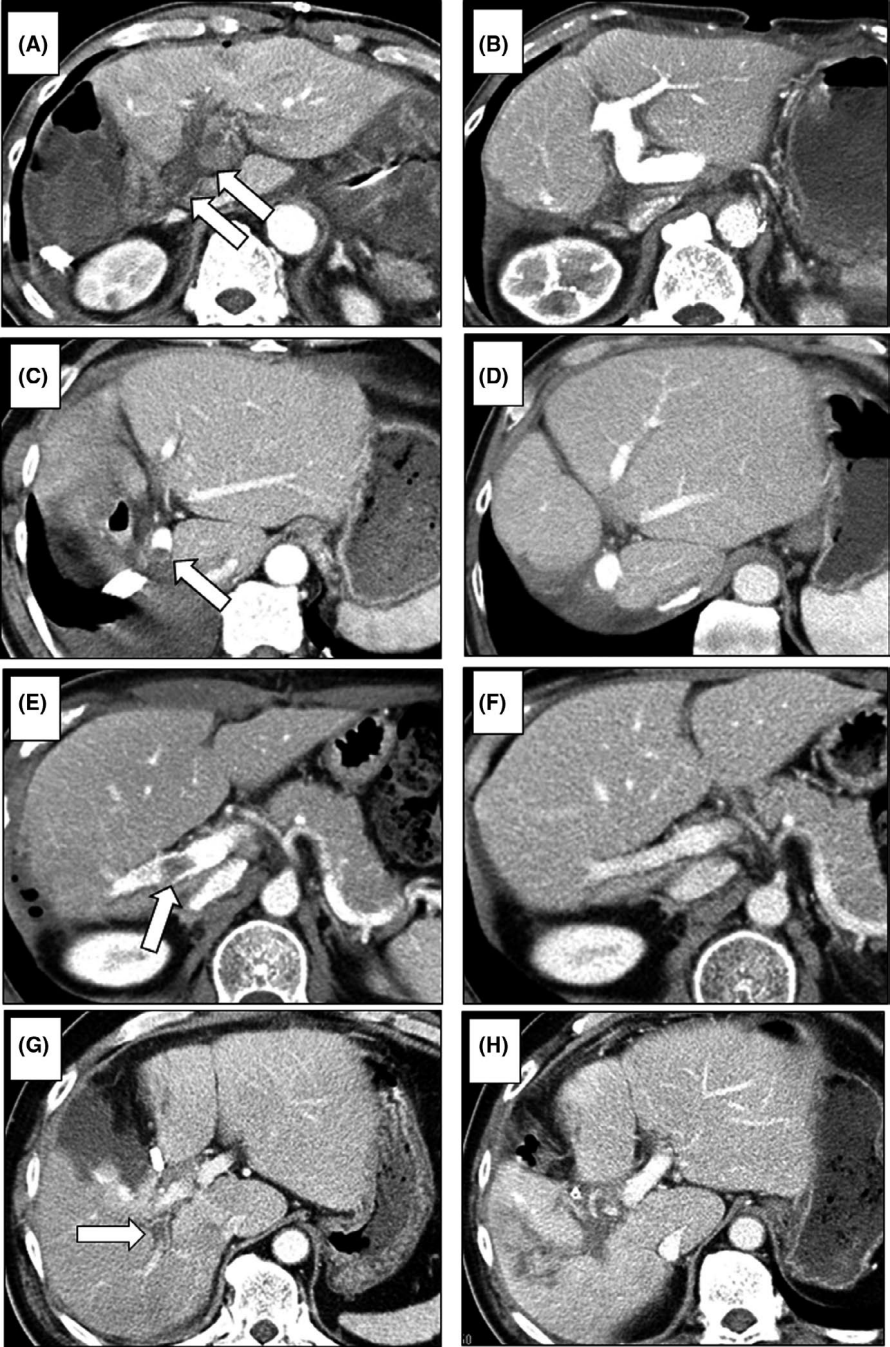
CT findings of PVT. In patients with complete obstruction of MPV thrombosis—main grade 3 (CT on POD 1) (arrows) (A)—the thrombus was removed by urgent surgical thrombectomy (CT on POD 21) (B). In patients with a small MPV thrombosis—main grade 1 (CT on POD 9) (arrow) (C)—the thrombus resolved without treatment (CT at 6 mo after surgery) (D). In patients with right‐branch PVT—hilar thrombosis (CT on POD 7) (arrow) (E)—the thrombus resolved with anticoagulation therapy (CT at 3 mo after surgery) (F). In patients with posterior branch PVT—hilar thrombosis (CT on POD 10) (arrow) (G)—the thrombus organized in spite of anticoagulation therapy (CT at 1 mo after surgery) (H)
